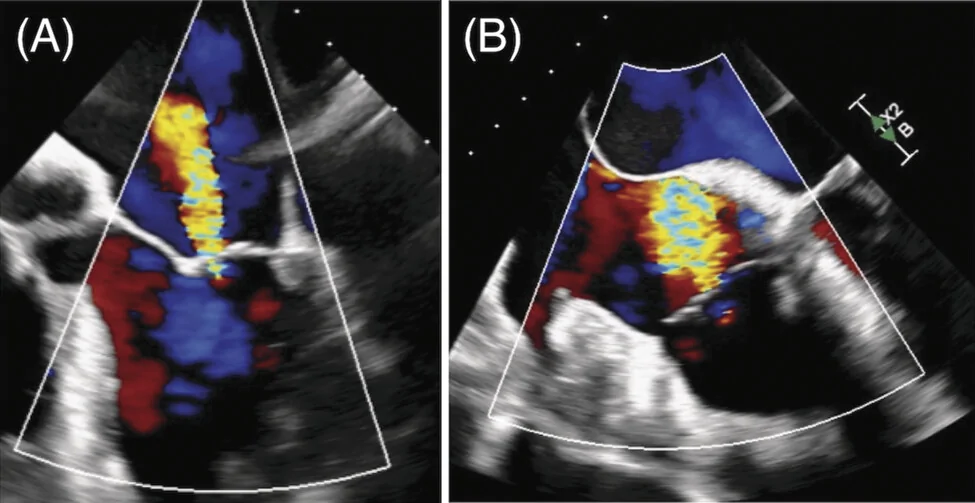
Preoperative mitral and tricuspid regurgitation. Transesophageal echocardiography demonstrating severe mitral regurgitation (
